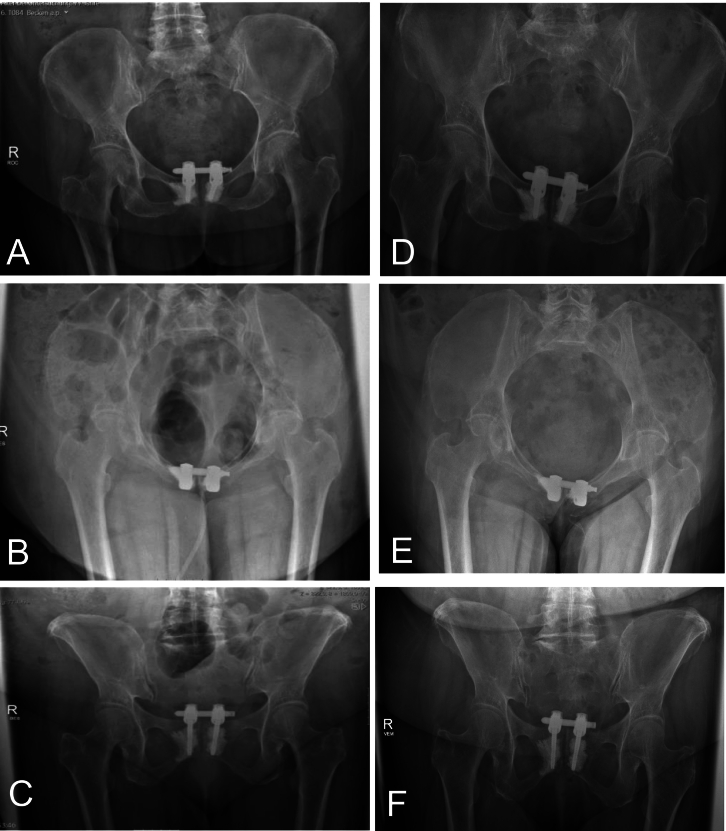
A-C Shows the postoperative X-ray, a.-p. (A), inlet (B) and outlet (C) view. D-F Shows the follow-up X-rays 7 years postoperative, a.-p. (D), inlet (E) and outlet (F) view.
